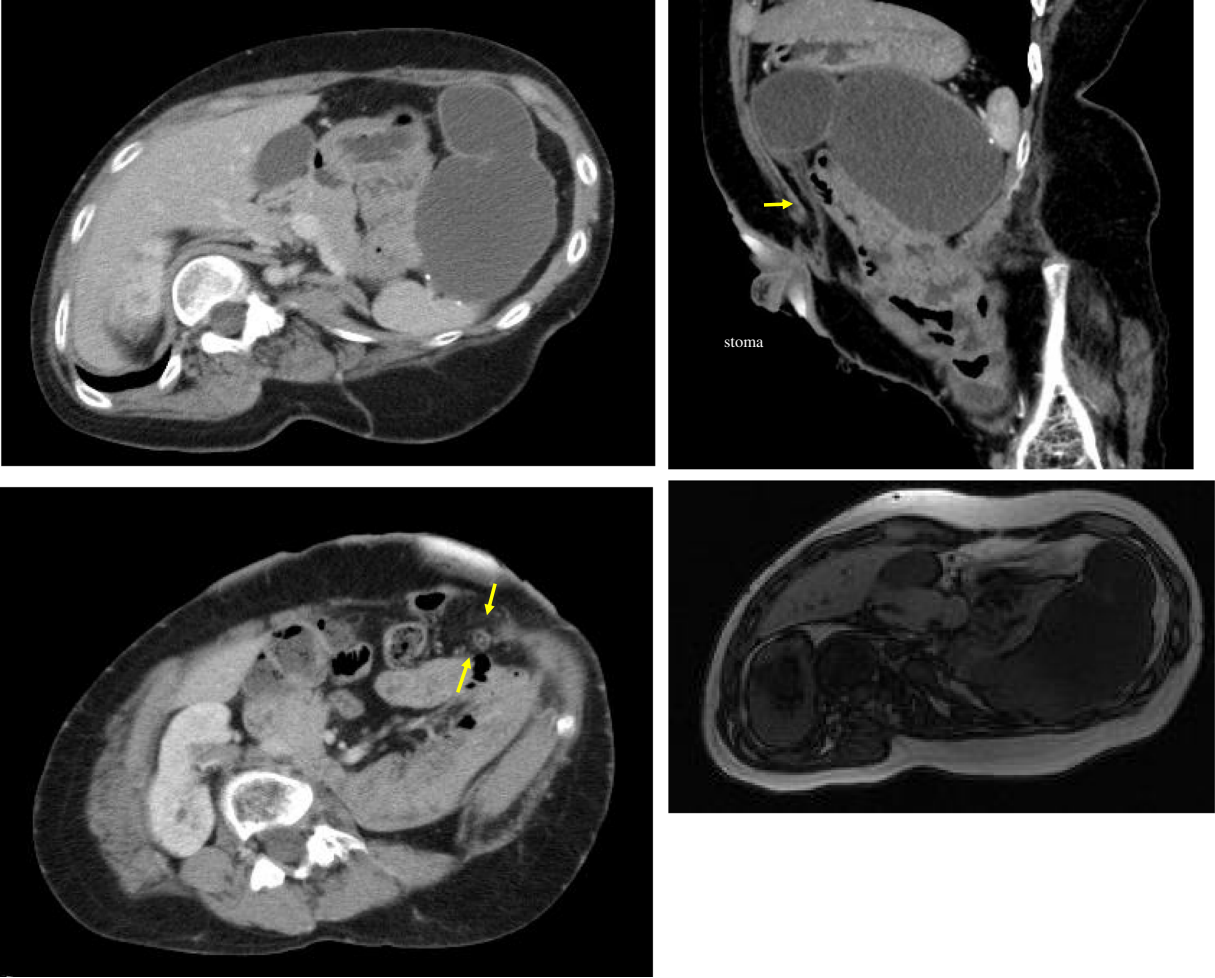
Contrast-enhanced CT image and MRI image of the tumor. Cystic and expansive tumor oriented between the pancreas, stomach, and spleen with no sign of invasion. The string-like structure interposed between the tumor and stoma. In the T1WI image, the cyst seemed to contain mucin and its different viscosities showed different strengths of MRI signals between the top and bottom of the cyst
